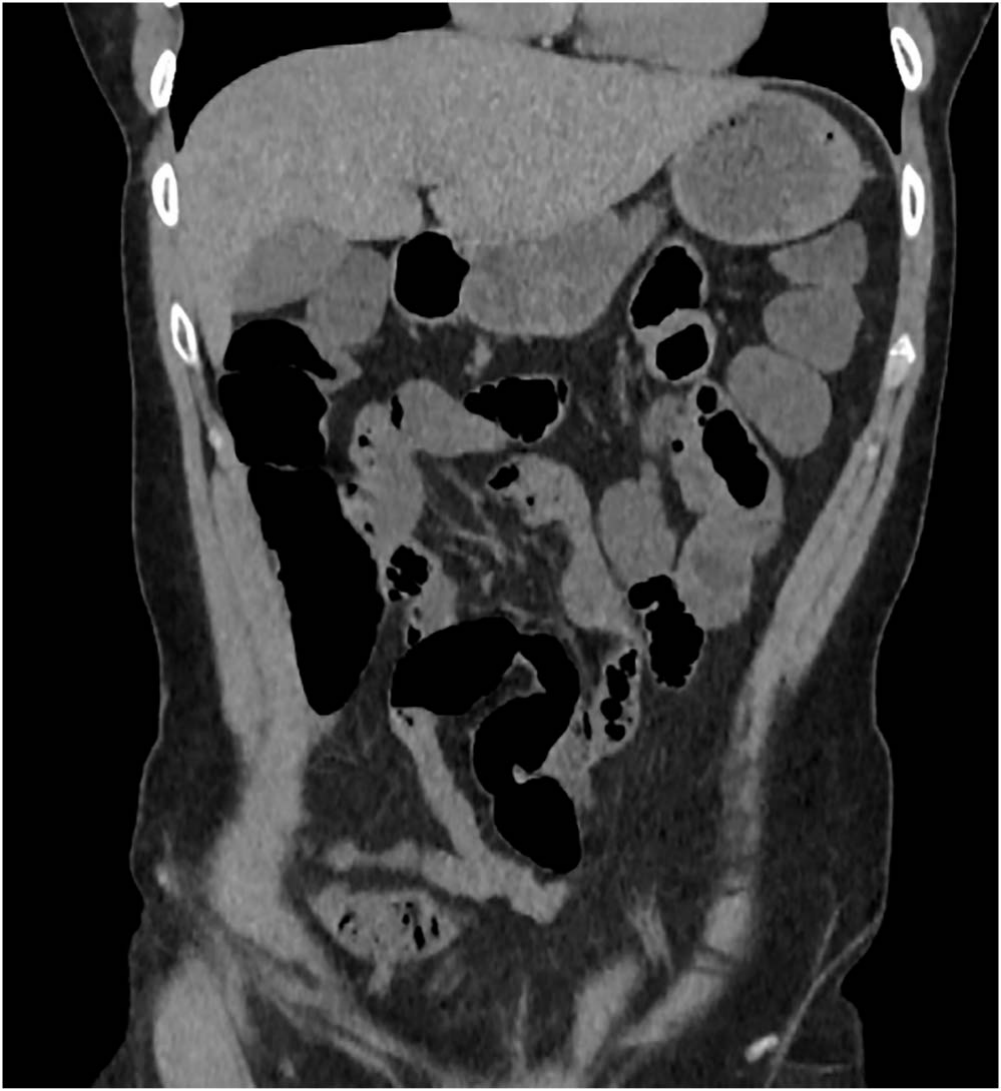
Abdominal CT showing dilated bowel loops with a transition point and no mechanical cause of obstruction.
